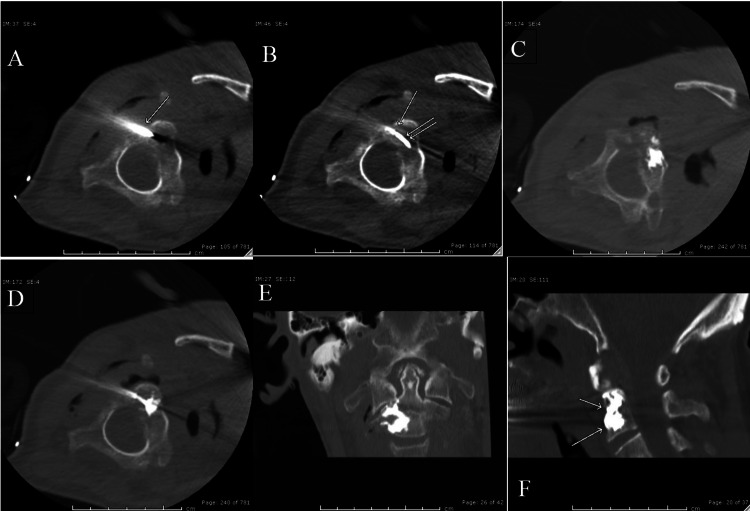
Vertebroplasty intra-procedural images Computed tomography (CT) images depicting the details of procedure. A) Initially, an 11 gauge outer cannula is inserted from the planned right posterolateral approach. B) A curved needle (double arrow) is introduced through the cannula (single arrow) in the lesion. The tip of needle placed further inferiorly and medially. C) Polymethyl methacrylate (PMMA) cement was injected in the inferior portion of the lesion. D) The curved needle was pulled back and reintroduced more superior-medially and further PMMA cement was injected. E) and F) demonstrate post-treatment distribution of PMMA cement in coronal and sagittal planes.
